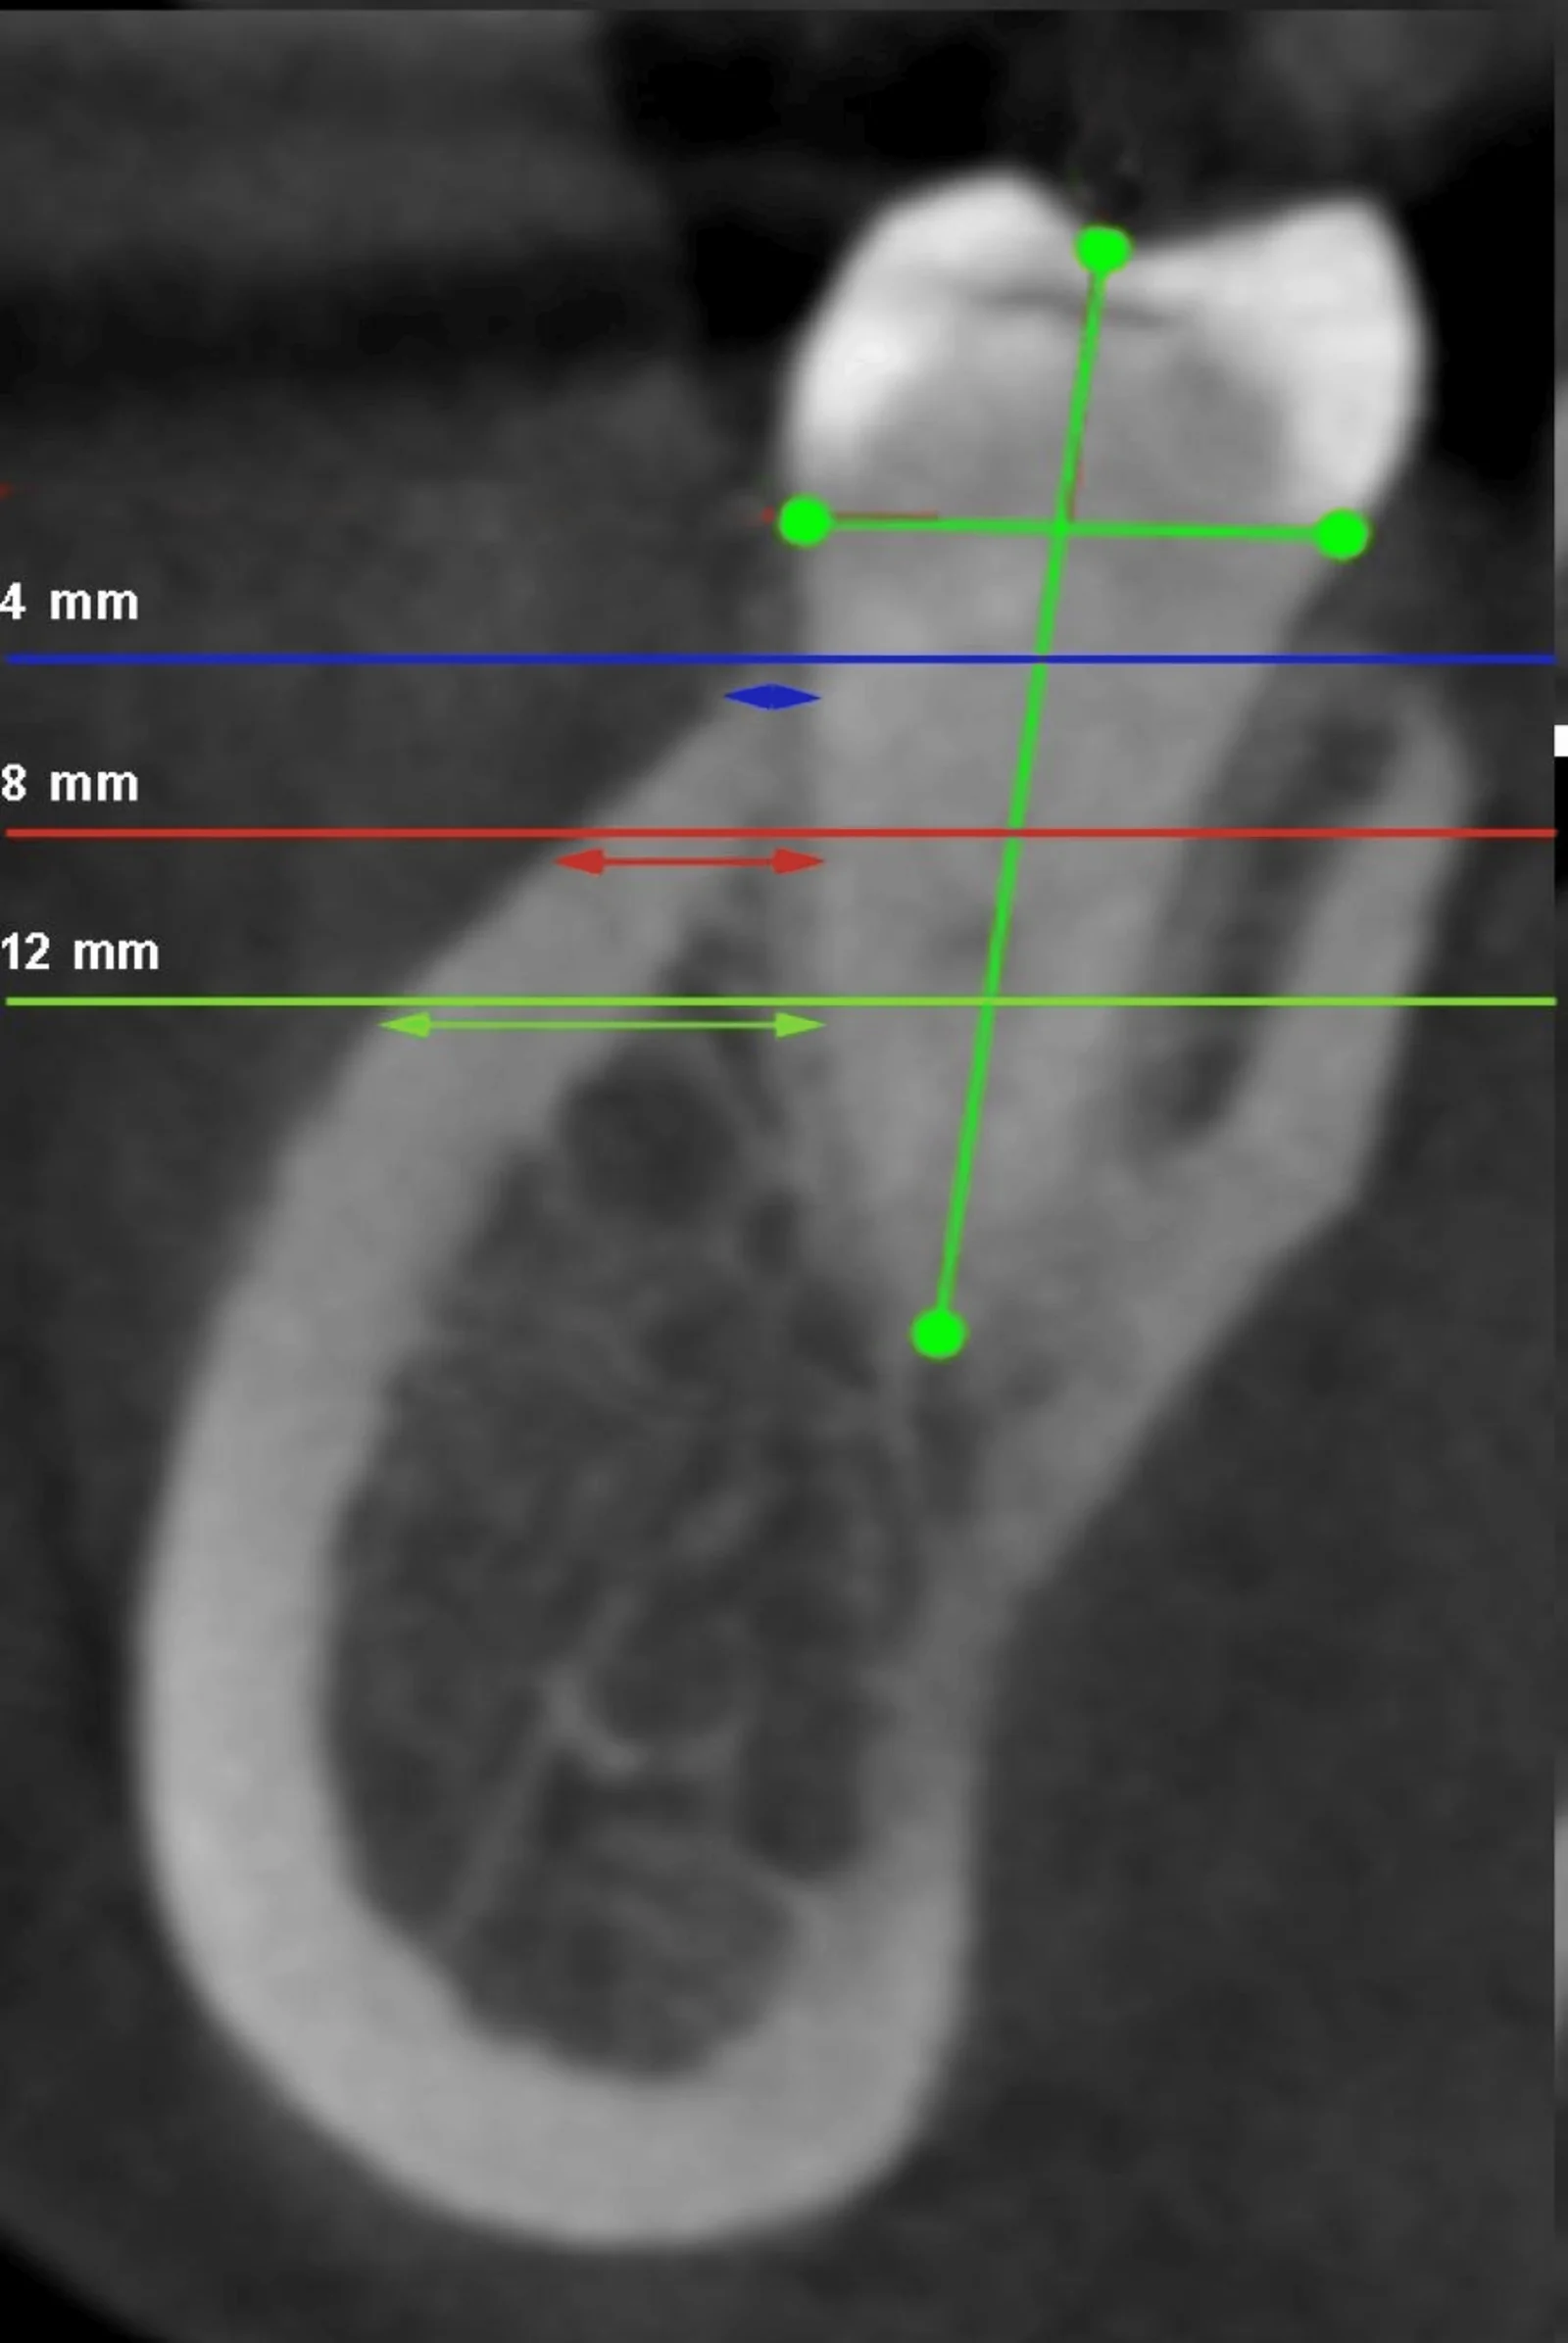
Cone beam computed tomography (CBCT) image of mandibular first molar showing bone thickness of buccal shelf area at 4, 8 and 12 mm distance from the cemento-enamel junction. Blue horizontal plane: 4 mm distance from cemento-enamel junction, Blue arrow: bone thickness of buccal shelf area. Red horizontal plane: 8 mm distance from cemento-enamel junction, Red arrow: bone thickness of buccal shelf area. Green horizontal plane: 12 mm distance from cemento-enamel junction, Green arrow: bone thickness of buccal shelf area. Original CBCT image of the patient from the study sample.
